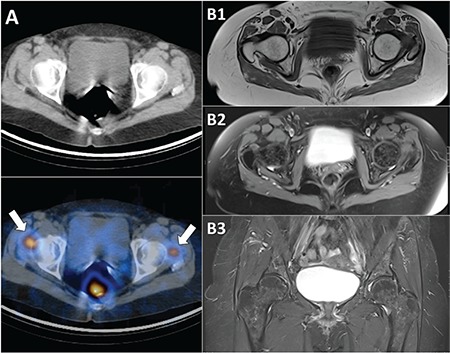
Similar to FDG-PET/CT (Figure 1 D), SPECT/CT images also showed focal uptakes on the soft tissues lateral to the femoral heads (A), which might be due to inflammation but the exact reason couldn’t be found because T1-weighted (B1) and fat-suppressed T2-weighted (B2, B3) images of magnetic resonance imaging were normal. Informed consent of the patient was obtained for all procedures. Many false-positive findings in I-131 scans due to physiological variants, inflammation or some non-thyroidal neoplasms have been reported (4,5,6,7,8). As a result, the following interesting/ rare situations were seen in combination in this case: false-positive I-131 uptakes at wedge-resection site and soft tissues, false-negative I-131 for FDGavid lymph metastasis, and detection of metastases after 12 years of disease-free follow-up that emphasizes the importance of long term follow-up. Our experience in this case also underlines the importance of careful interpretation of WBS. Focal I-131 uptake at the pulmonary wedge-resection site could be a false-positive finding due to focal traction bronchiectasis. Diagnostic CT should be performed to clarify this suspicious finding in order to determine if there is a residual lesion.
